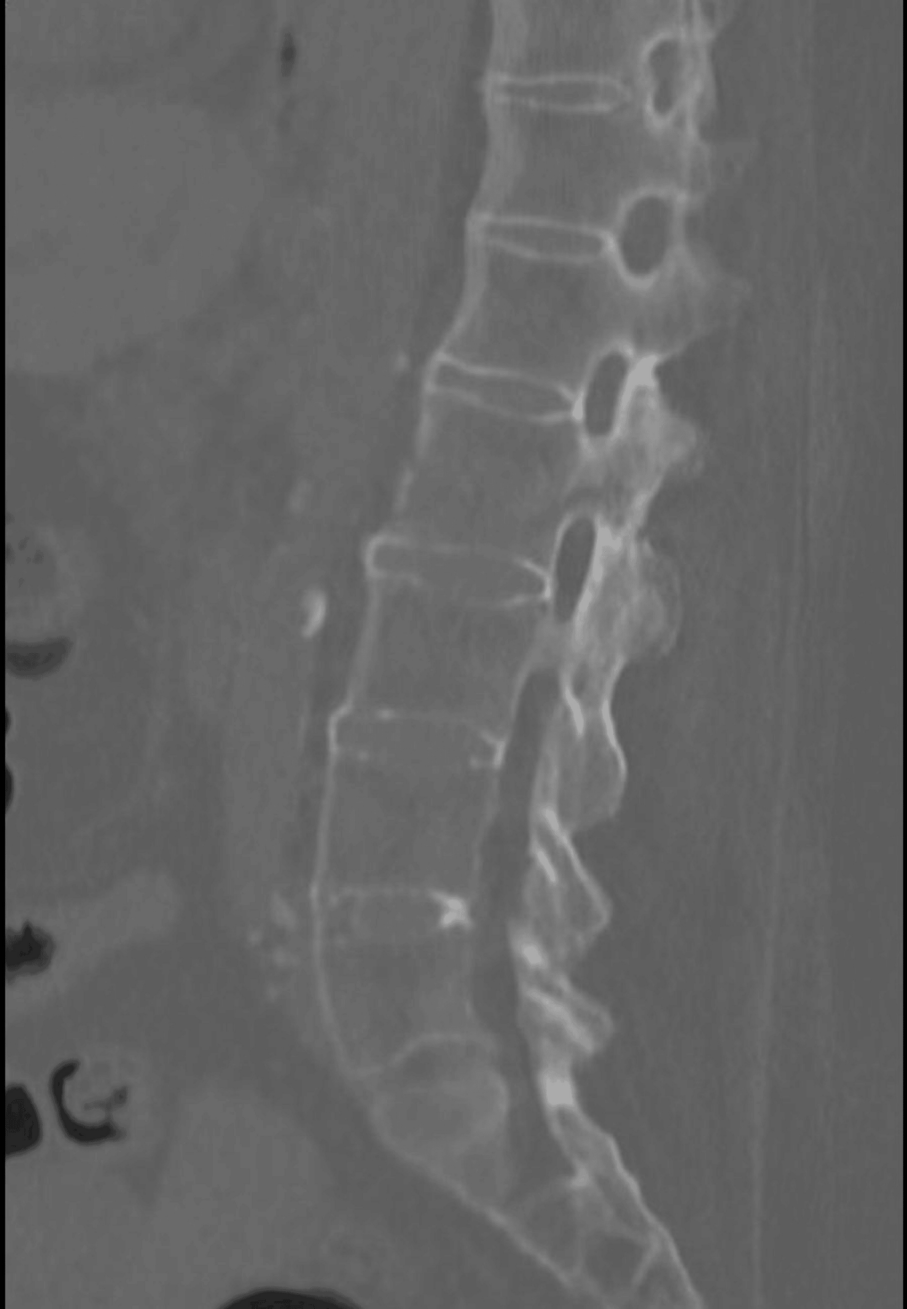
Preoperative CT of L2 fracture Sagittal CT image showing an unstable L2 chalk-stick fracture prior to surgical intervention.
